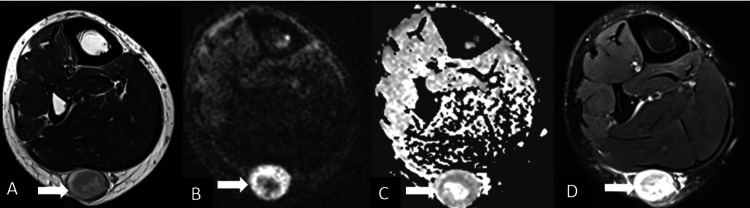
MRI of the calf showing neurofibroma MRI of the calf region in a 30-year-old male with progressively increasing swelling of the right calf for four months showing a well-defined lesion in the subcutaneous plane in the posterior aspect of the leg. The lesion is heterogeneously hyperintense on T2-weighted images with a thin peripheral hypointense rim (A) with no significant diffusion restriction (B, C) and moderate to strong heterogeneous contrast enhancement (D). Diagnosis: neurofibroma.
